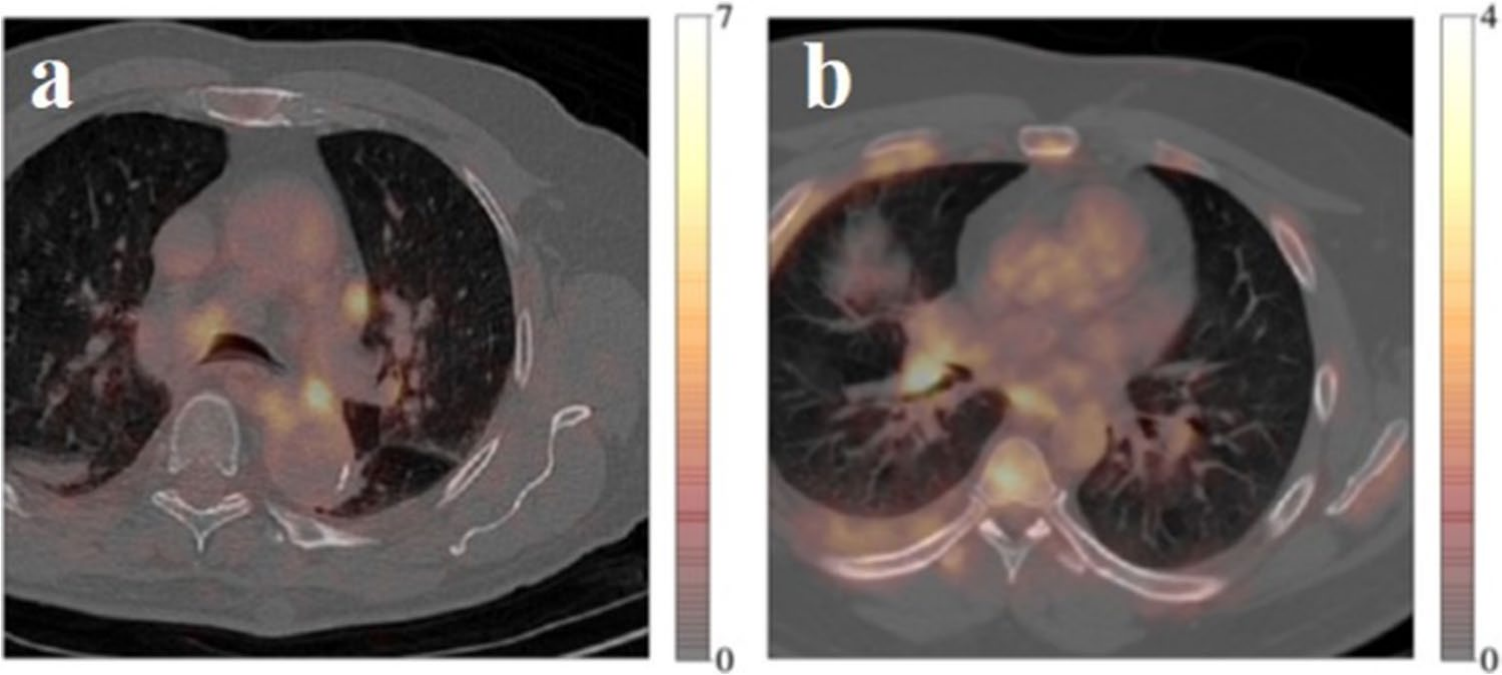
A comparison of [18F]FDG-PET/CT images from a scanner 1, Gemini TF (Philips Medical Systems), and b scanner 2, Discovery MI (GE Medical Systems)
